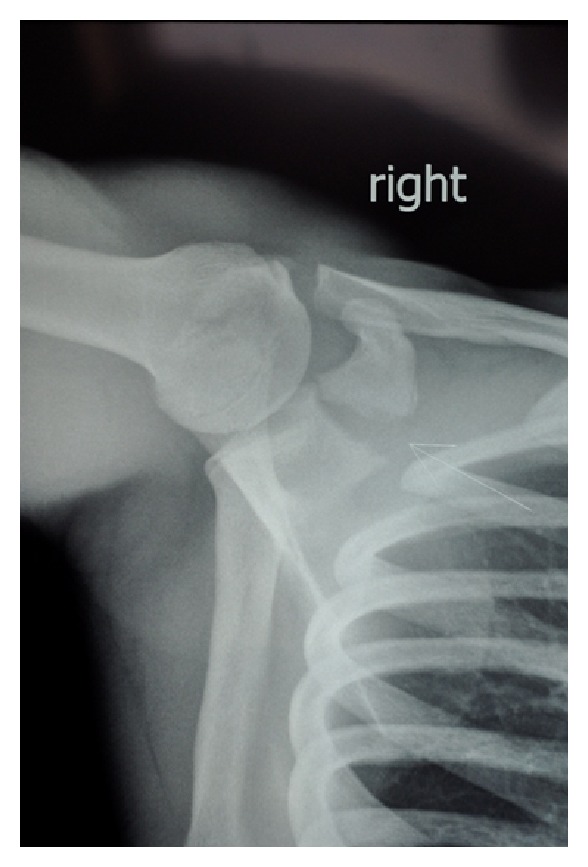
Case A: initial axillary radiograph.
